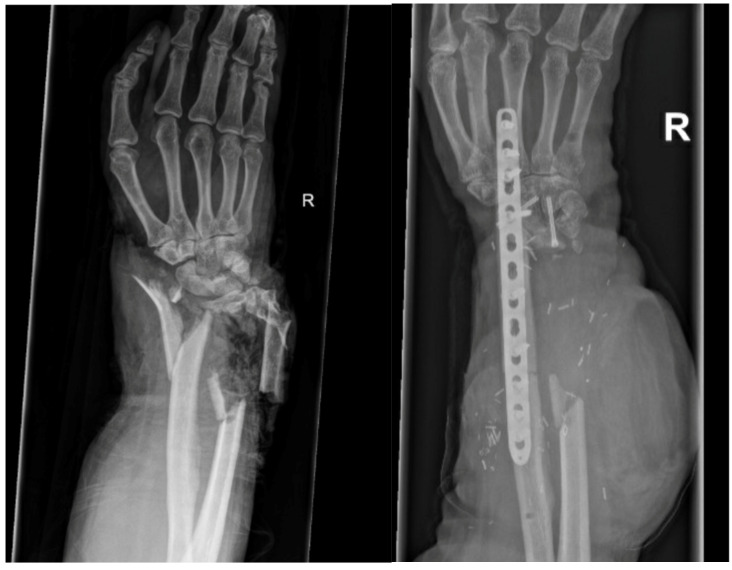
Initial X-ray after trauma (left) and 2 months after reconstruction with free fibula and wrist fusion (right).
